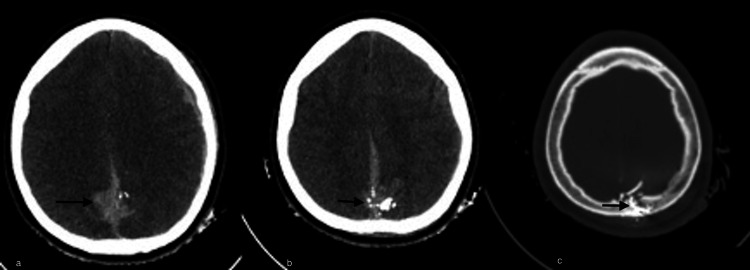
Admission plain skull computed tomography. (a) The image on the left shows bilateral parieto-occipital subarachnoid hemorrhage (arrow), and the image in the center (b) (arrow), and on the right depicts intra and extracranial projectiles (c) (arrow).
